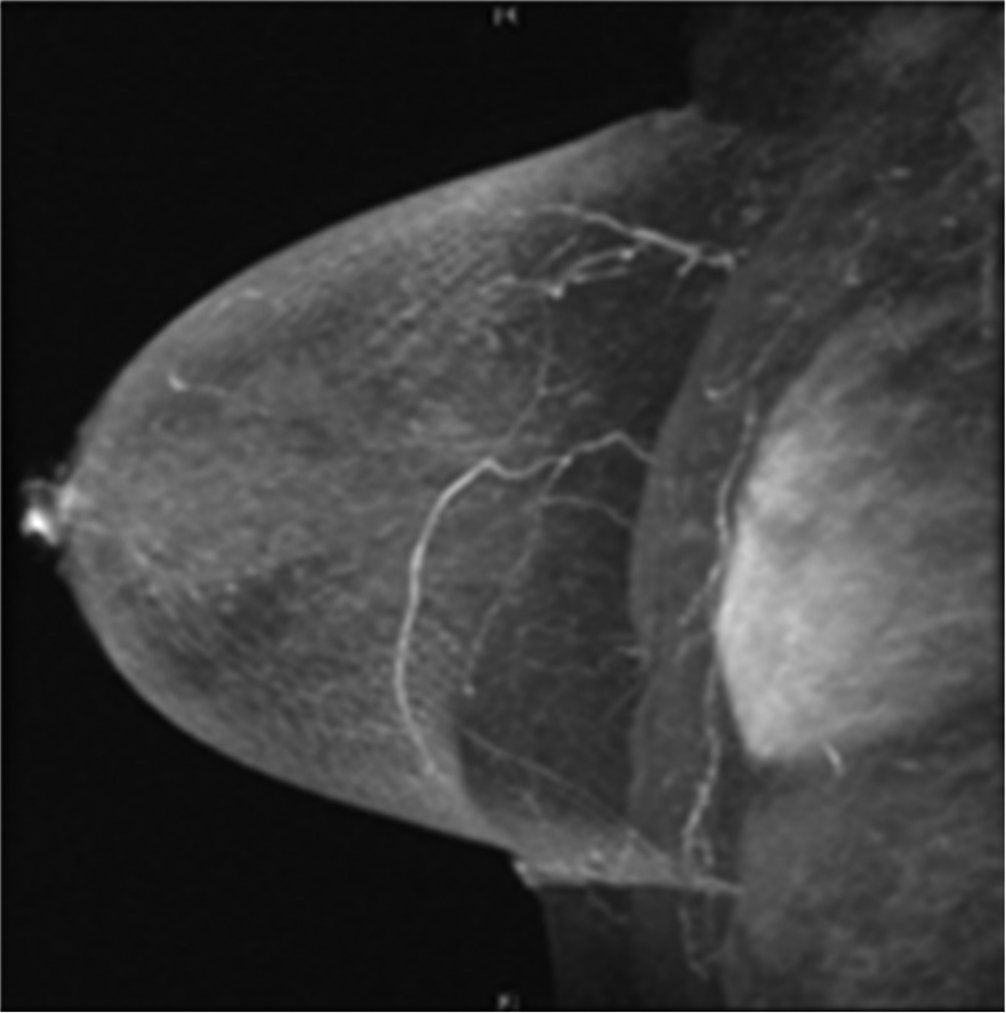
3D maximum intensity projection reconstructed sagittal post-contrast fat-suppressed *T*_1_ weighted MRI of the left breast performed after the completion of neoadjuvant chemotherapy demonstrating no residual lesion.
